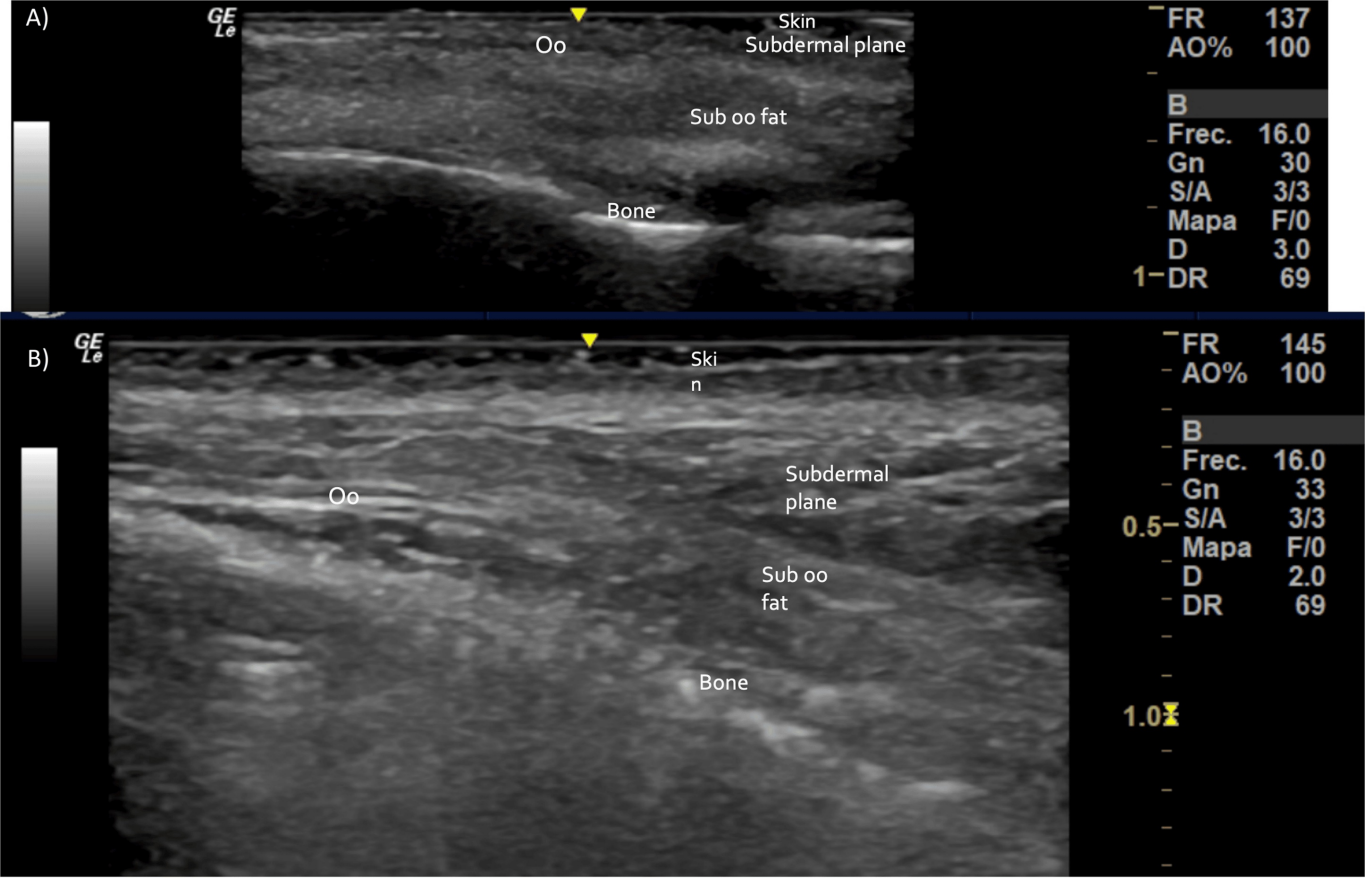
Skin and soft tissue high-resolution ultrasound, immediately after treatment: a slight increase in tissue echogenicity is visualized in all the tissue planes. Images are shown using the medial-view (A) and lateral-view (B).
